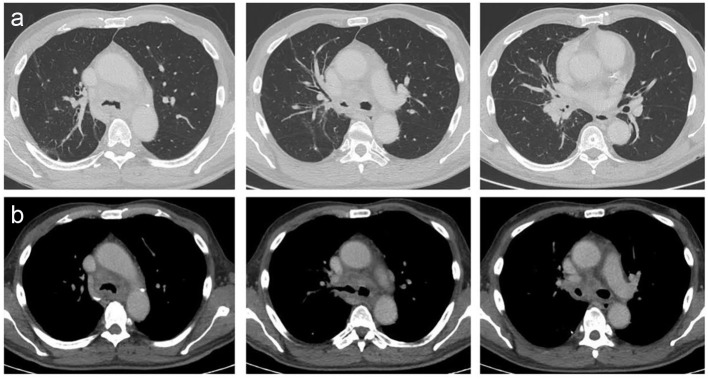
Tomographic images. (a) Normal lung parenchyma observed in different cuts of the HRCT. Commitment in the tracheal lumen with a narrowness in the right bronchus source due to an hilar mass. (b) Mediastinal windows observed in the HRCT. Tracheal bulge and adenomegalies in the mediastinum and hilum were observed.
